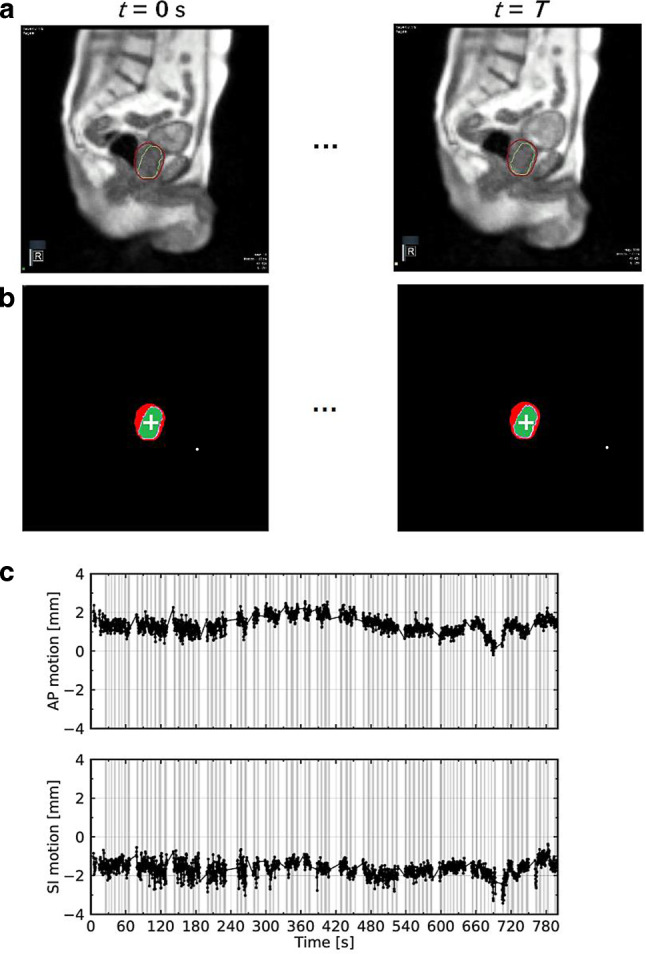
Extraction of the centroid motion of the target contour in anterior-posterior and superior–inferior directions from the cine MRI data for a typical fraction (patient 1, fraction 3). a Cine MRI frames at the beginning (*t* = 0 s) and the end (*t* = *T*) of the fraction. Target (*green*) and boundary (*red*) contours are overlaid on the MR image. The coloured square in the left lower corner shows the beam status (*green*: beam off, *yellow*: beam on). b Contour filling and extraction of the centroid position of the target (*white cross*) and the boundary. c Obtained motion curve of the moving target centroid relative to the static boundary centroid (*grey shaded area*: beam-on phases). Interruptions in the curves are due to imaging pauses during gantry rotation
